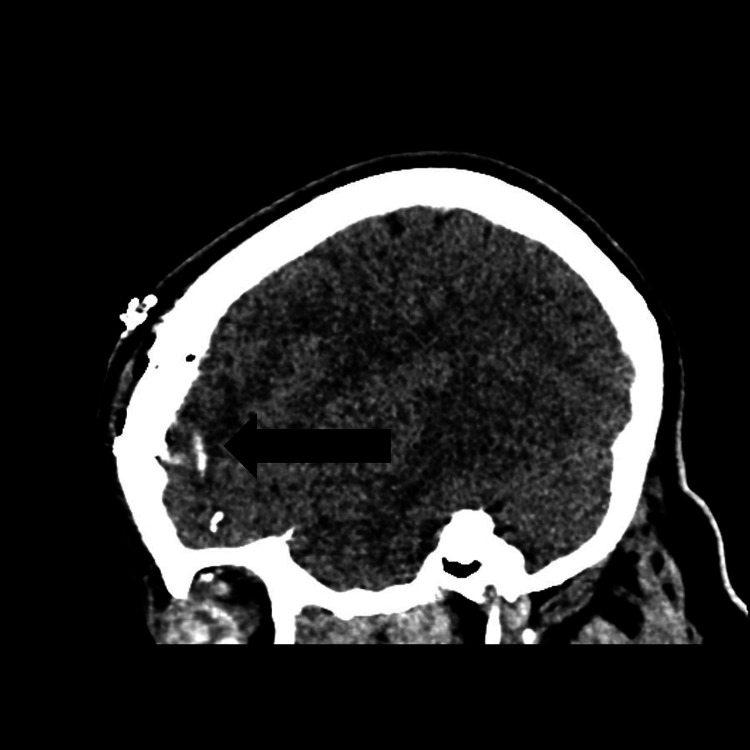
Postoperative sagittal CT showing residual hematoma after foreign body removal CT: computed tomography Postoperative sagittal CT image demonstrates residual hematoma along the track of the removed foreign body, confirming successful extraction and highlighting the area of localized hemorrhage
